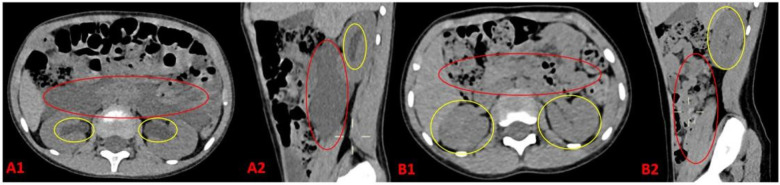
Postoperative follow-up imaging of a representative case. (A) Preoperative CT scans (axial, A1; sagittal, A2) show a large cystic lesion in the retroperitoneum (red circle) accompanied by bilateral hydronephrosis (yellow circle). (B) Postoperative CT scans at 3 months (axial, B1; sagittal, B2) demonstrate complete resolution of both the cystic lesion (red circle) and the bilateral hydronephrosis (yellow circle).
